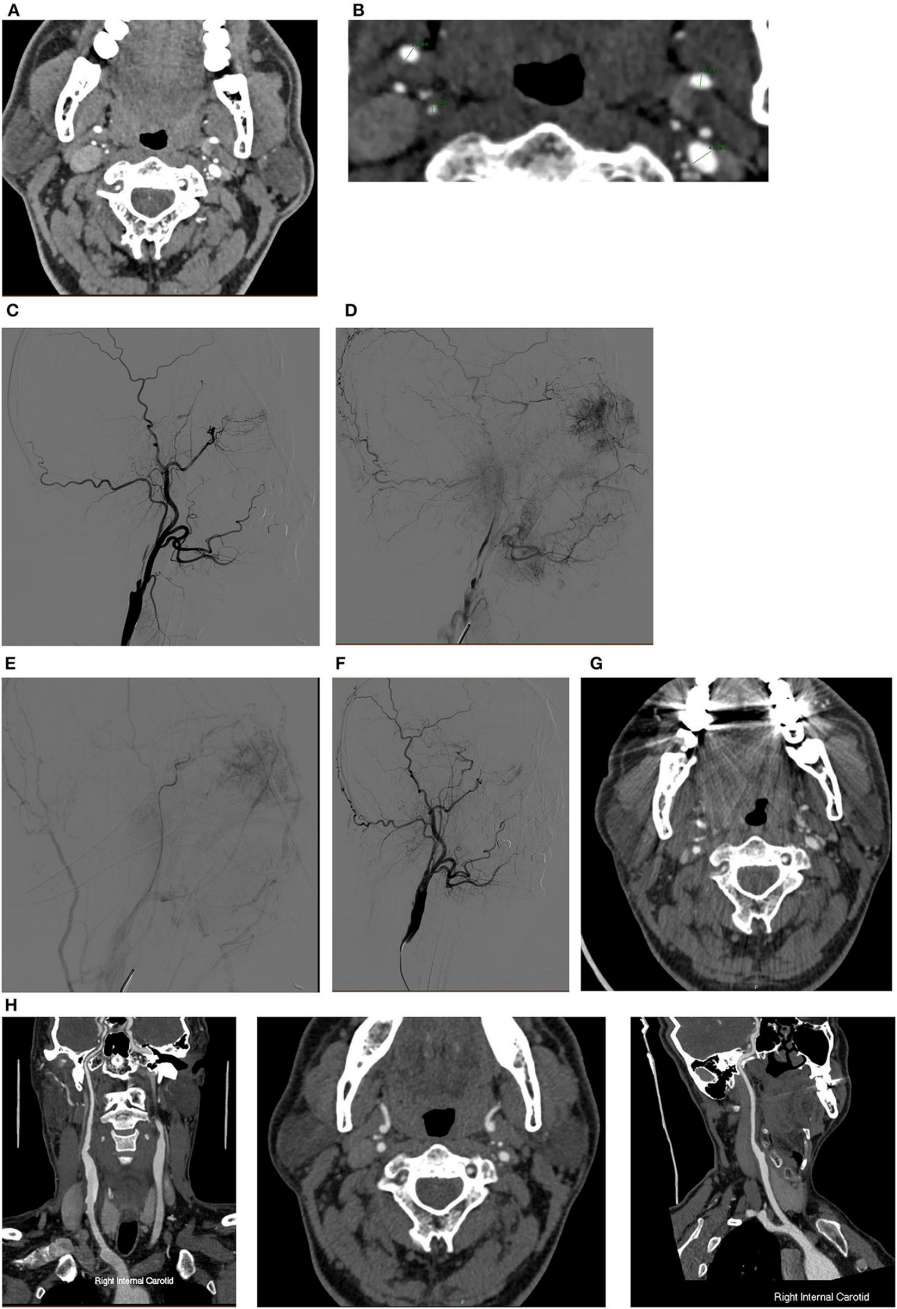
A typical case of CEA for CNO with distal full collapse. (A,B) CTA characteristics for CNO with distal collapse. Right ICA: 1.51 mm, right ECA: 2.86 mm, left ICA: 2.69 mm, left ECA: 4.31 mm, right ICA/left ICA: 0.56 ( ≤ 0.87), right ICA/ECA: 0.53 (≤1.27); DSA characteristics for CNO with distal collapse. (C) Severe stenosis of the ICA with a collapsed distal ICA; (D) delayed time of contrast arrival of distal ICA; (E) delayed antegrade flow of the patent ICA in the late arterial phase; (F) consistent contrast arrival of ICA and ECA after CEA. The 30-day and 12-month CTA follow-up showed the RICA is patent. (G) Axial and sagittal view of 30-day CTA; (H) axial and sagittal view of 12-month CTA.
